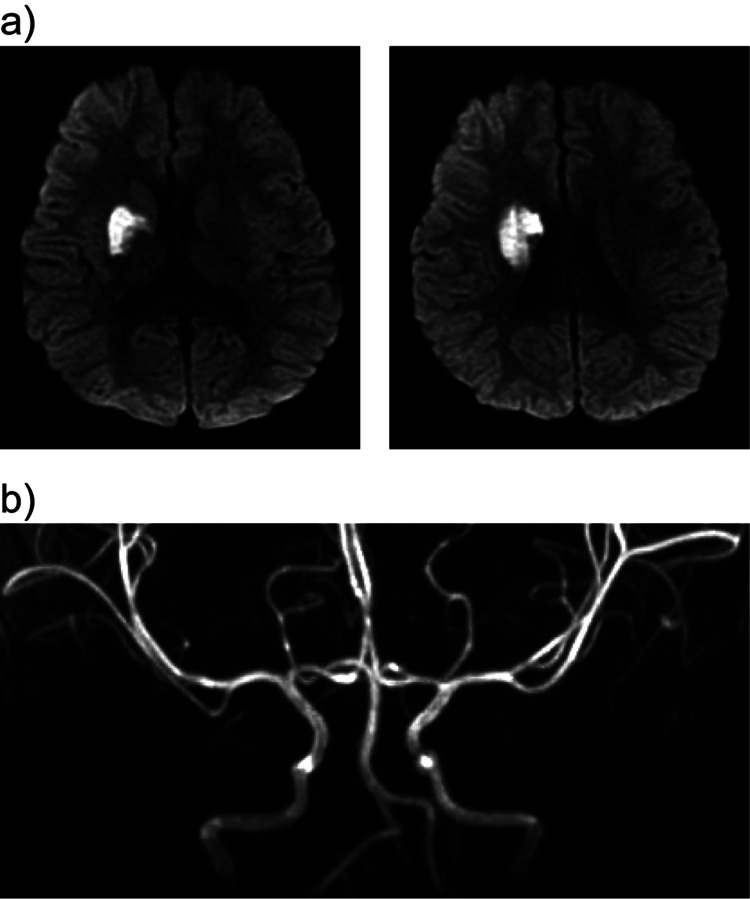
Head Magnetic Resonance Imaging (MRI) with diffusion-weighted imaging (DWI) and Magnetic Resonance Angiography (MRA) on day two. a) A high signal was detected at the right nucleus basalis in DWI. b) An MRA showed no obvious abnormalities.
